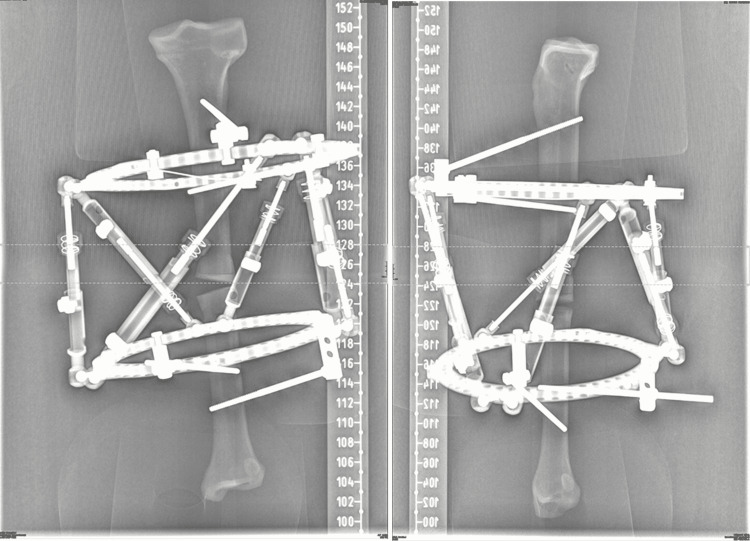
Post-correction radiograph of the tibia as an example
